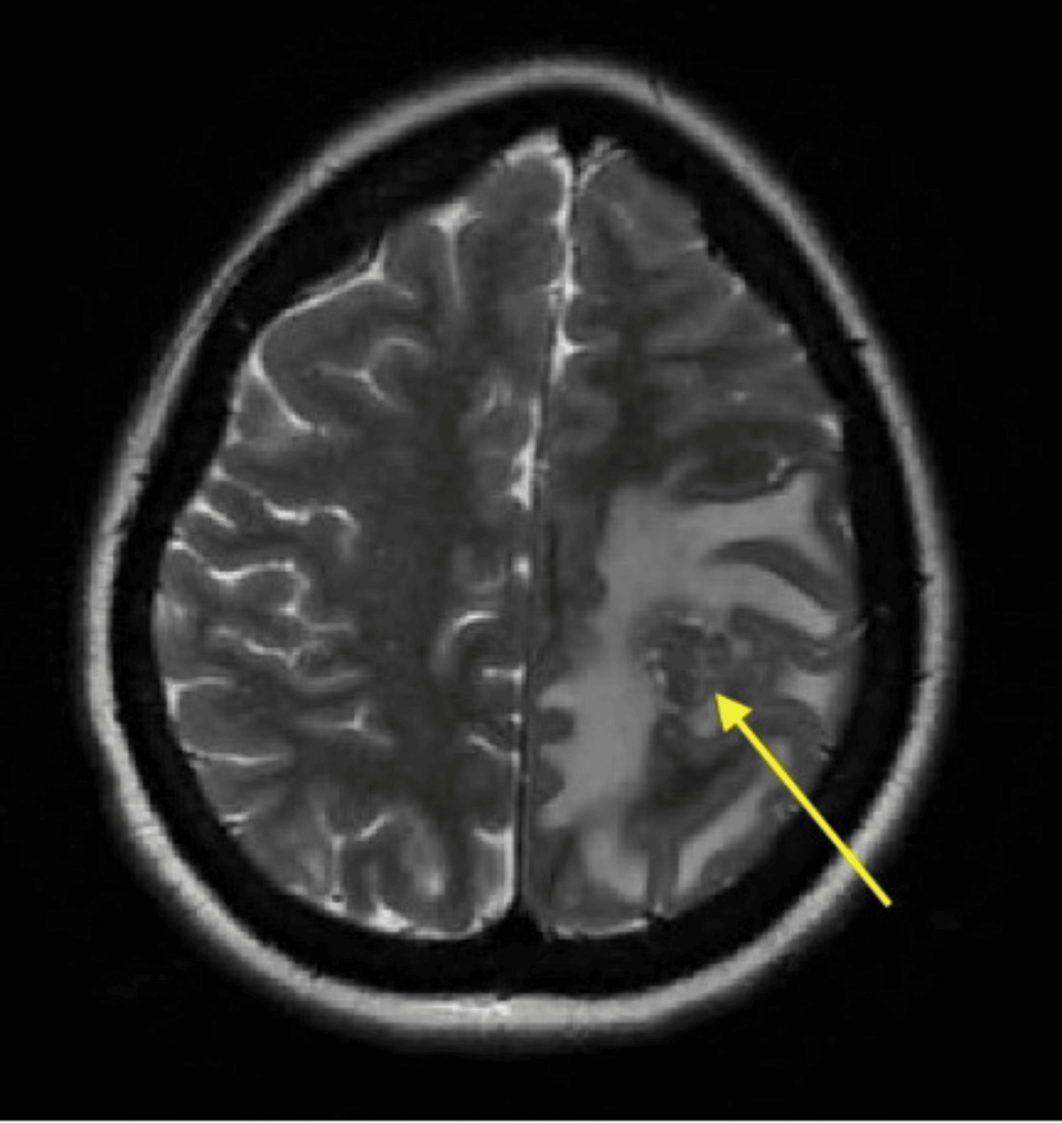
Axial T2-weighted MRI demonstrating a lesion in the left parietal lobe (yellow arrow) accompanied by surrounding vasogenic edema.
